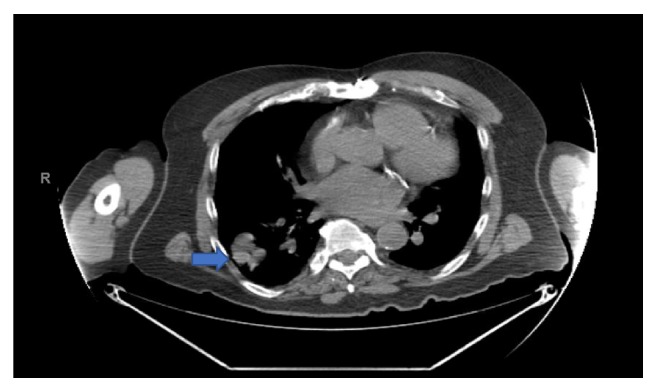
PET scan. Arrow: moderate to intensely increased metabolic activity in the right lower lobe mass (max SUV 8.4 initially increasing to 15.5 on delayed images), without evidence of other focal abnormalities.
